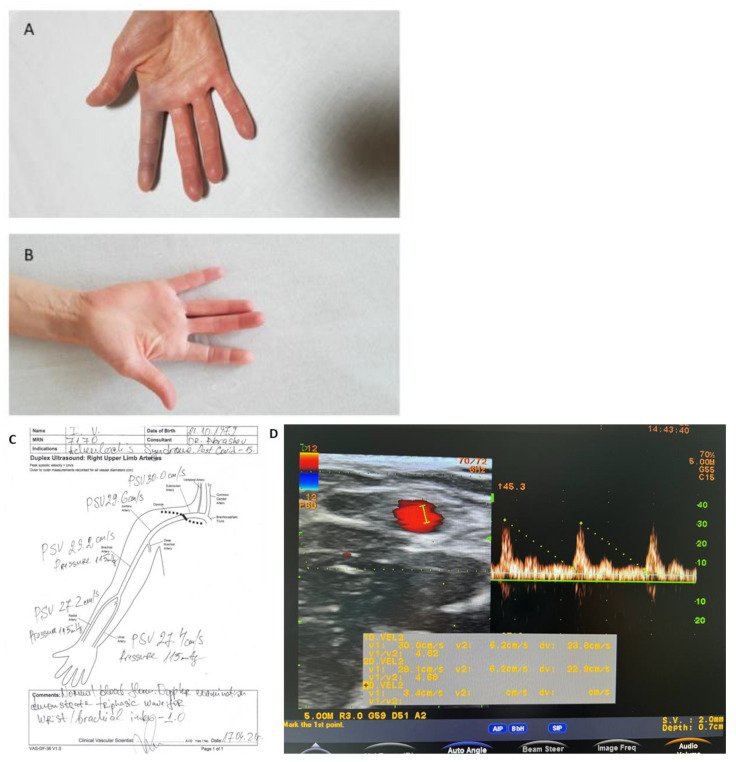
(A,B). A 43-year-old woman presented with AS symptoms including sudden pain, bruising, and edema on her right index finger. Hand pictures: (A) the first day on admission; and (B) the first day on discharge; (C,D). Data of Doppler investigation (C) and Doppler ultrasound result on the first day (D) showed normal peak forward velocities in cm/s. Doppler examination demonstrates triphasic waveforms. Brachial/wrist index of 1.0.
